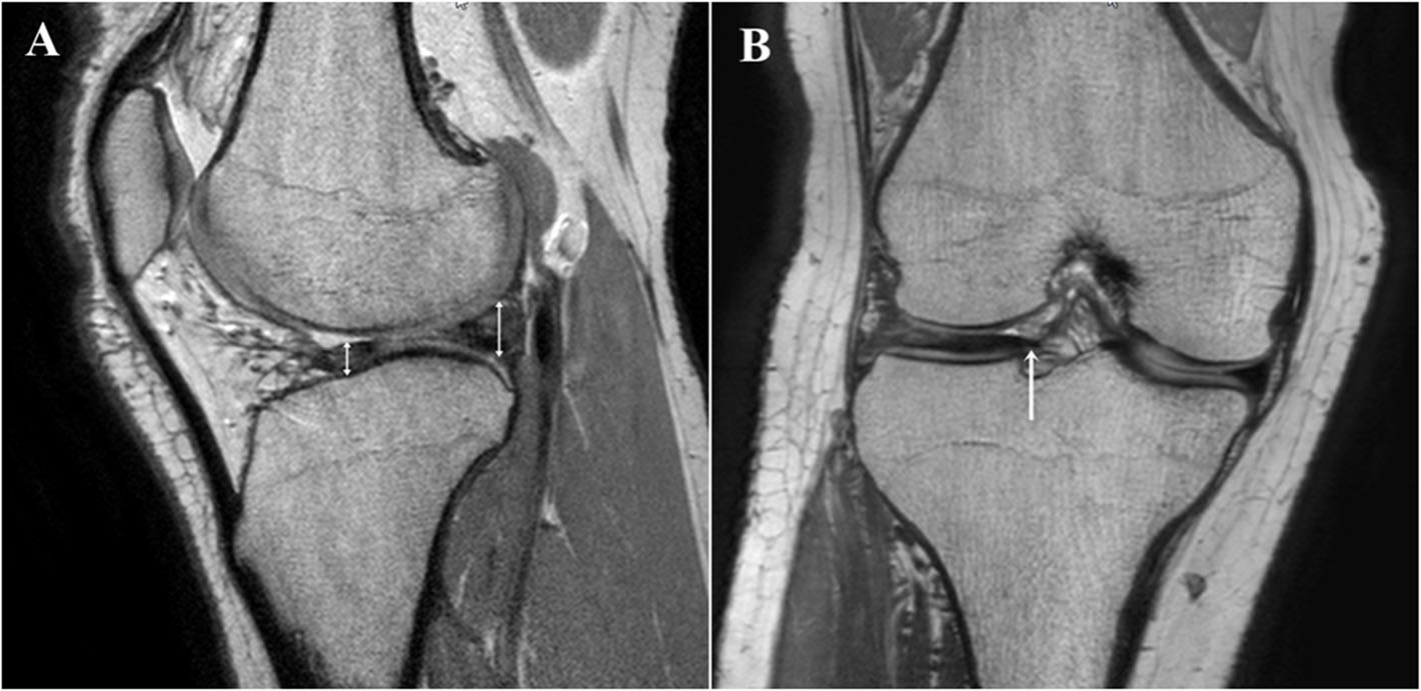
Deformation of discoid lateral meniscus on MRI. (A) the meniscus shows a greater than 70% difference of anteroposterior thickness in sagittal image (*two sided arrows*: thickness of meniscus). (B) the meniscal segment crosses the lateral tibial spine in coronal image (*arrow*)
